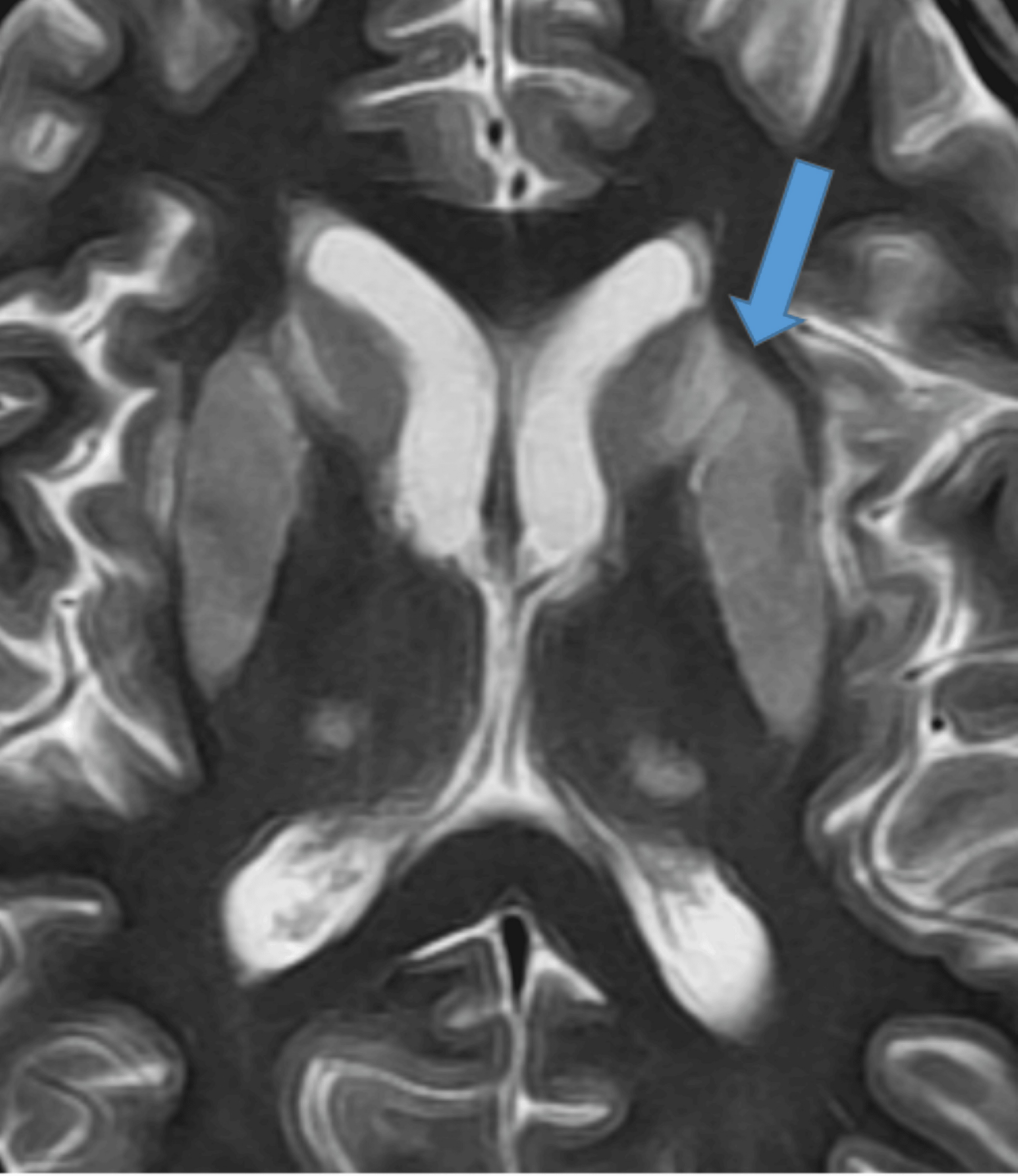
T2-Weighted Axial MRI Sequence Showing Hyperintense Signal (Blue Arrow). MRI: magnetic resonance imaging
